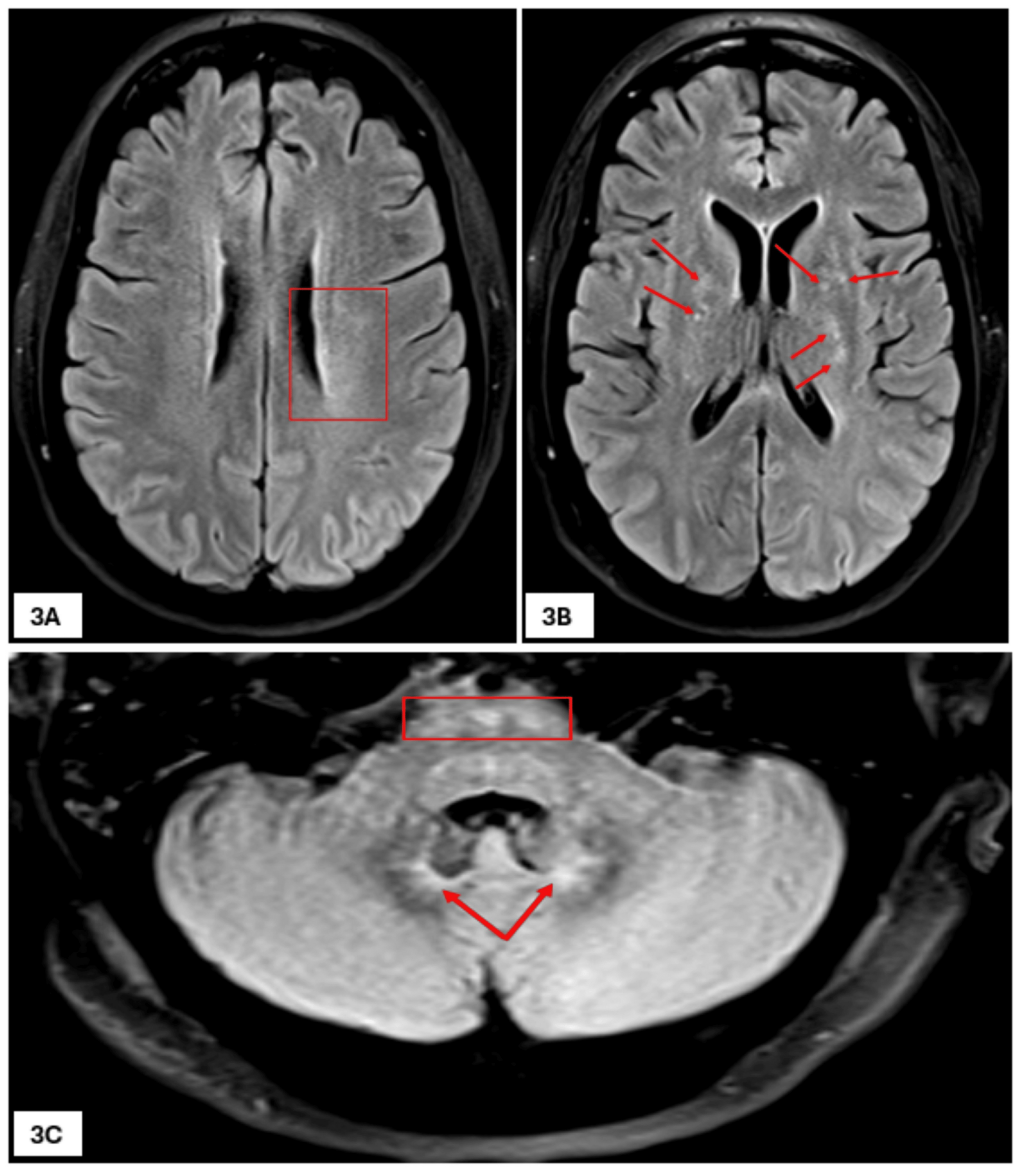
Second MRI scan of the brain. Two-dimensional axial FLAIR images showing progressive changes - bilateral deep periventricular white matter with indistinct areas of FLAIR signal alteration (rectangle in 3A); basal ganglia foci of elevated FLAIR signal (arrows in 3B); indistinct foci in the pontine tegmentum (rectangle in 3C); and the ventral aspects of both dentate nuclei (arrows in 3C). FLAIR: fluid-attenuated inversion recovery
